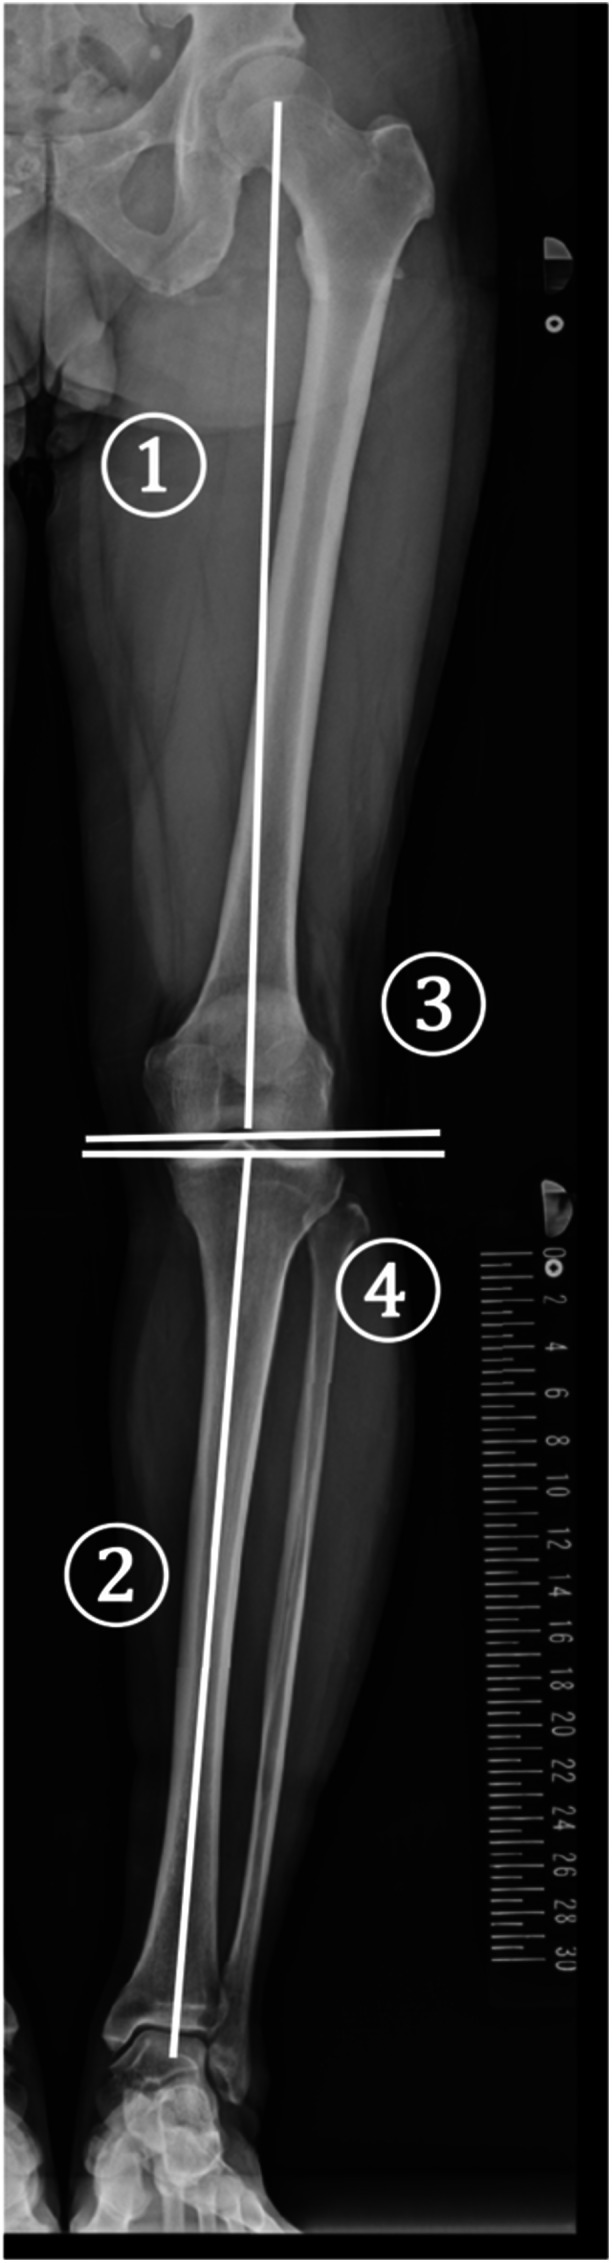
Radiographic assessment. The standing full‐length lower extremity was used for the measument. Each of the lines was drawn as follows: (1) Mechanical axis of the femur. (2) Mechanical axis of the tibia. (3) Tangent to the femoral condyle. (4) Tangent to the tibial plateau. The hip–knee–ankle angle (HKA), lateral distal femoral angle (LDFA), and medial proximal tibial angle (MPTA) correspond to the angles defined by lines (1) and (2), (1) and (3), and (2) and (4), respectively. Knee joint line obliquity (KJLO) corresponds to the angle defined by line (4) and the horizontal line.
